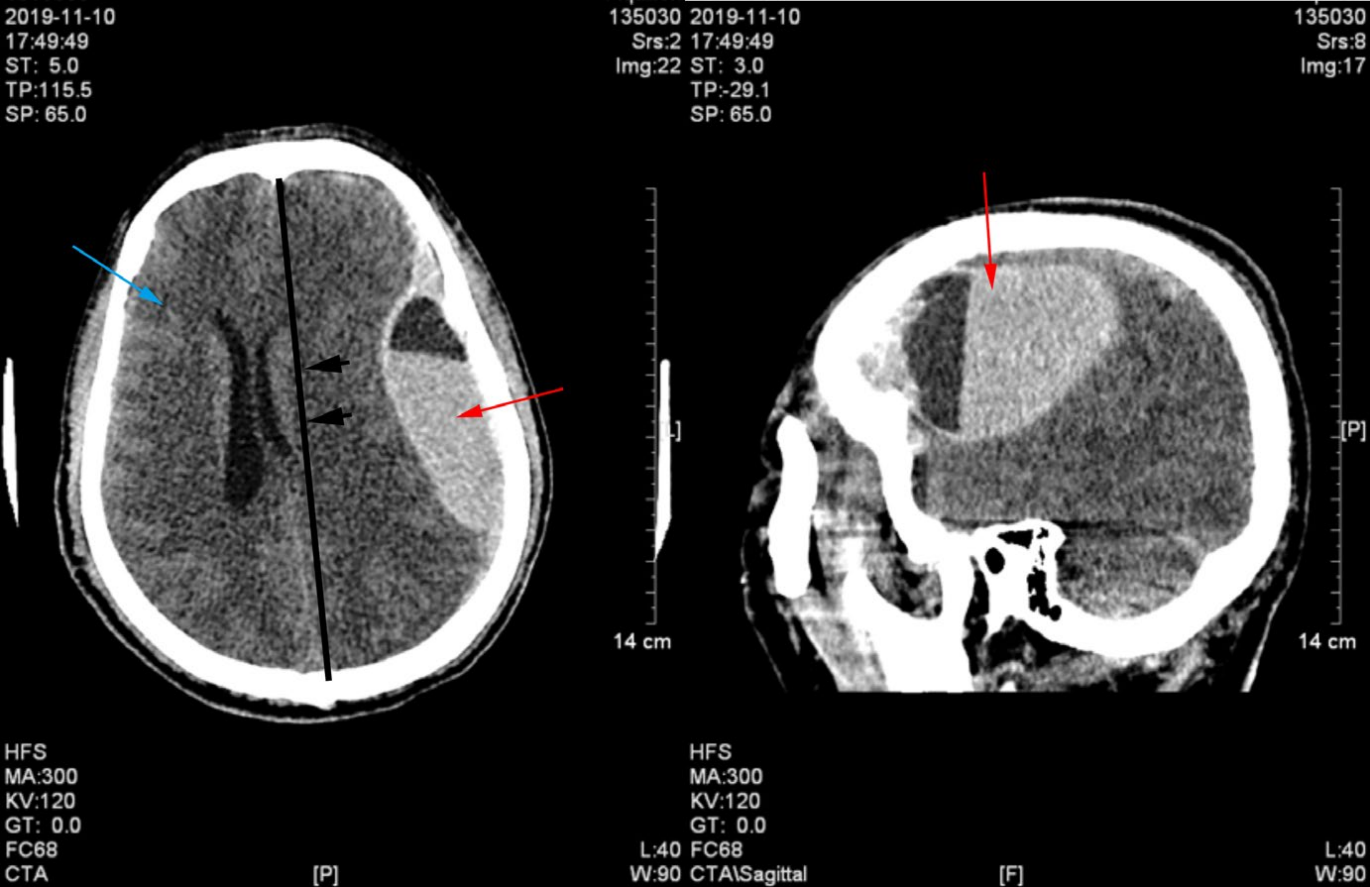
Head CT on admission indicated subdural hematoma (red arrow), brain infarction (blue arrow), and subfalcine herniation (dark arrow)
